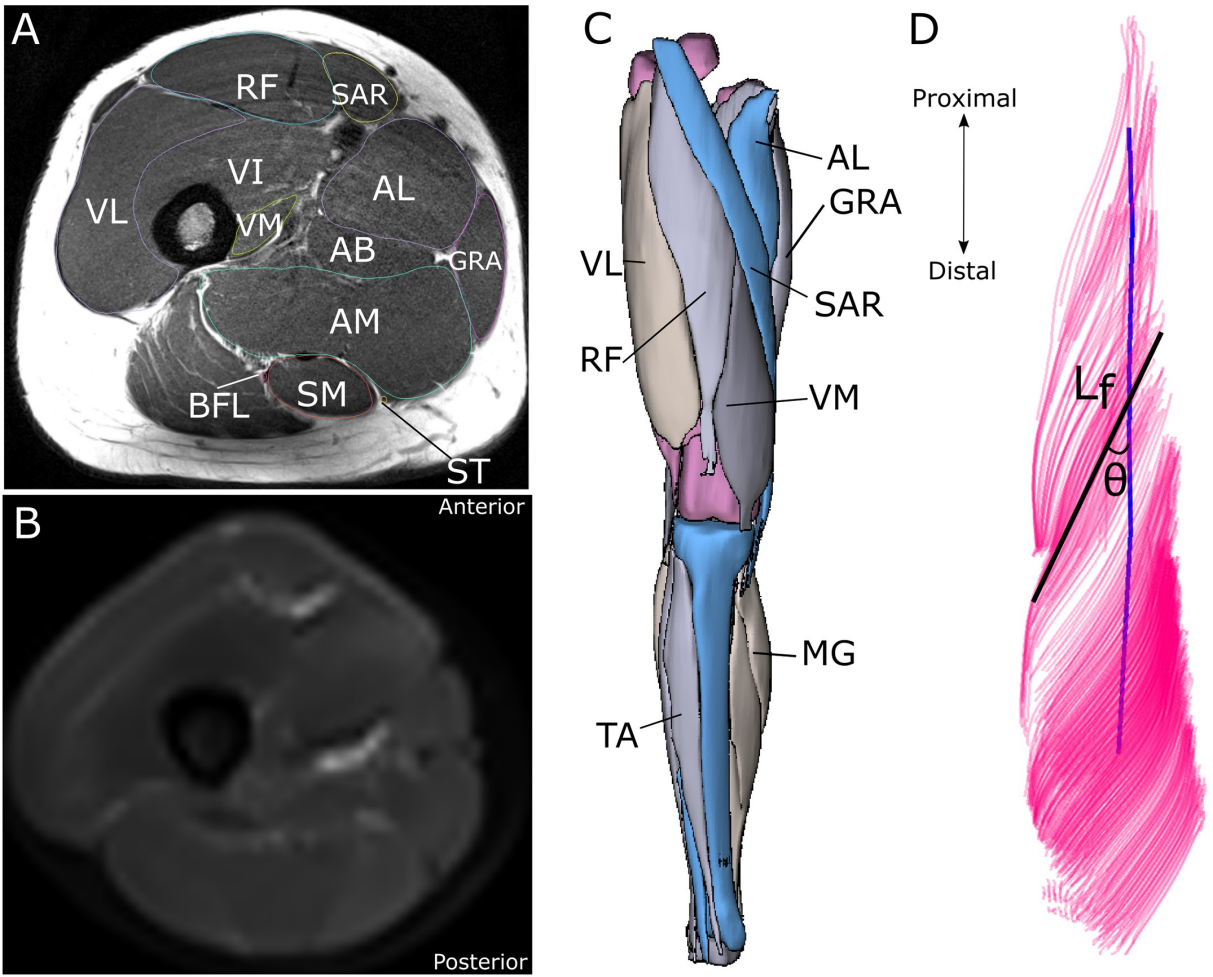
Representative T1-weighted MR anatomical image (A) and diffusion tensor image (B) of the thigh segment of one subject. Muscles and bones were digitally segmented from the T1 images to create 3D representations of the lower limbs (C) (for muscle abbreviation definitions, see Table 2). Muscle fascicles (fibers) were tracked from the diffusion weighted MR images (D). From these 3D point cloud-based models, it was possible to measure fiber length (L_f_) and surface fiber pennation angle (θ, angle of the fibers relative to the muscle’s line of action (blue line)).
